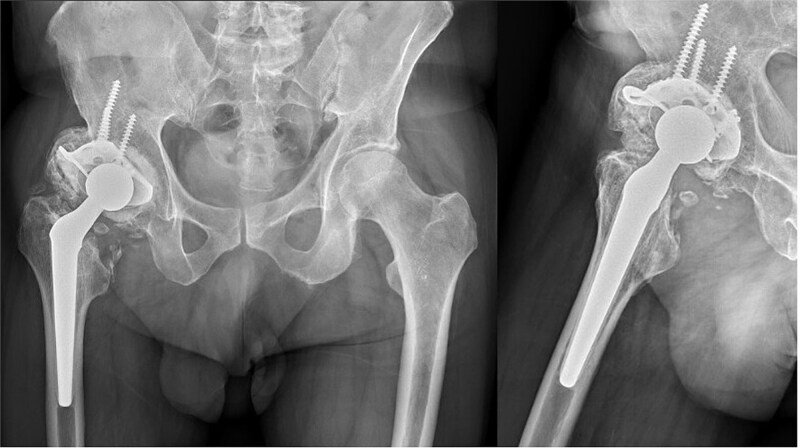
Postoperative THA radiographs at 16-year follow-up (2026) confirming complete autograft incorporation and well-fixed components. Despite moderate acetabular wear and mature heterotopic bone, no signs of implant loosening or instability are present.
